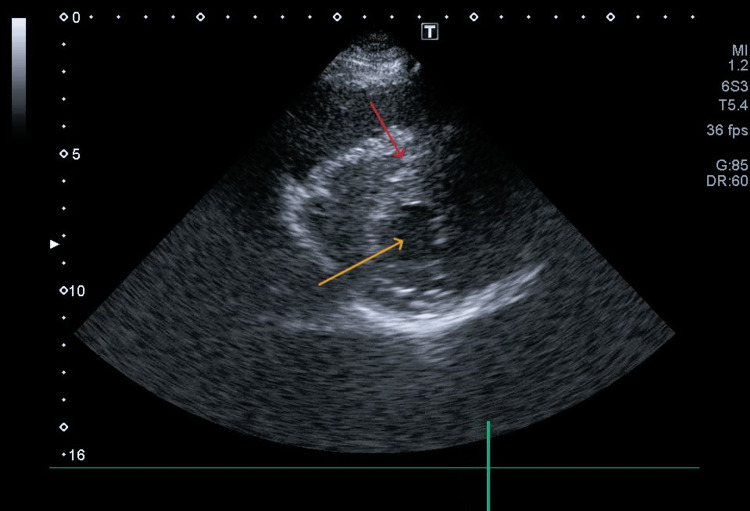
Marked reduction of the left ventricular cavity due to septal hypertrophy Parasternal short-axis transthoracic echocardiography view demonstrating extreme hypertrophy of the interventricular septum (red arrow), with marked reduction of the left ventricular cavity (yellow arrow).
